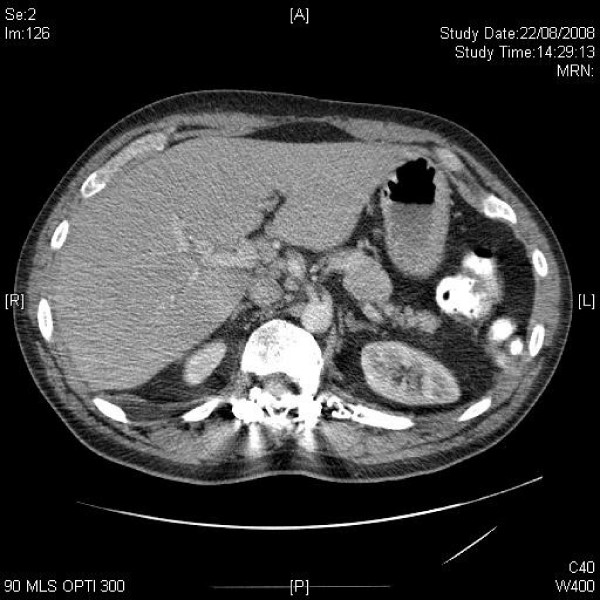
Axial CT of upper abdomen demonstrates mildly enlarged para-aortic and portal lymph nodes.
